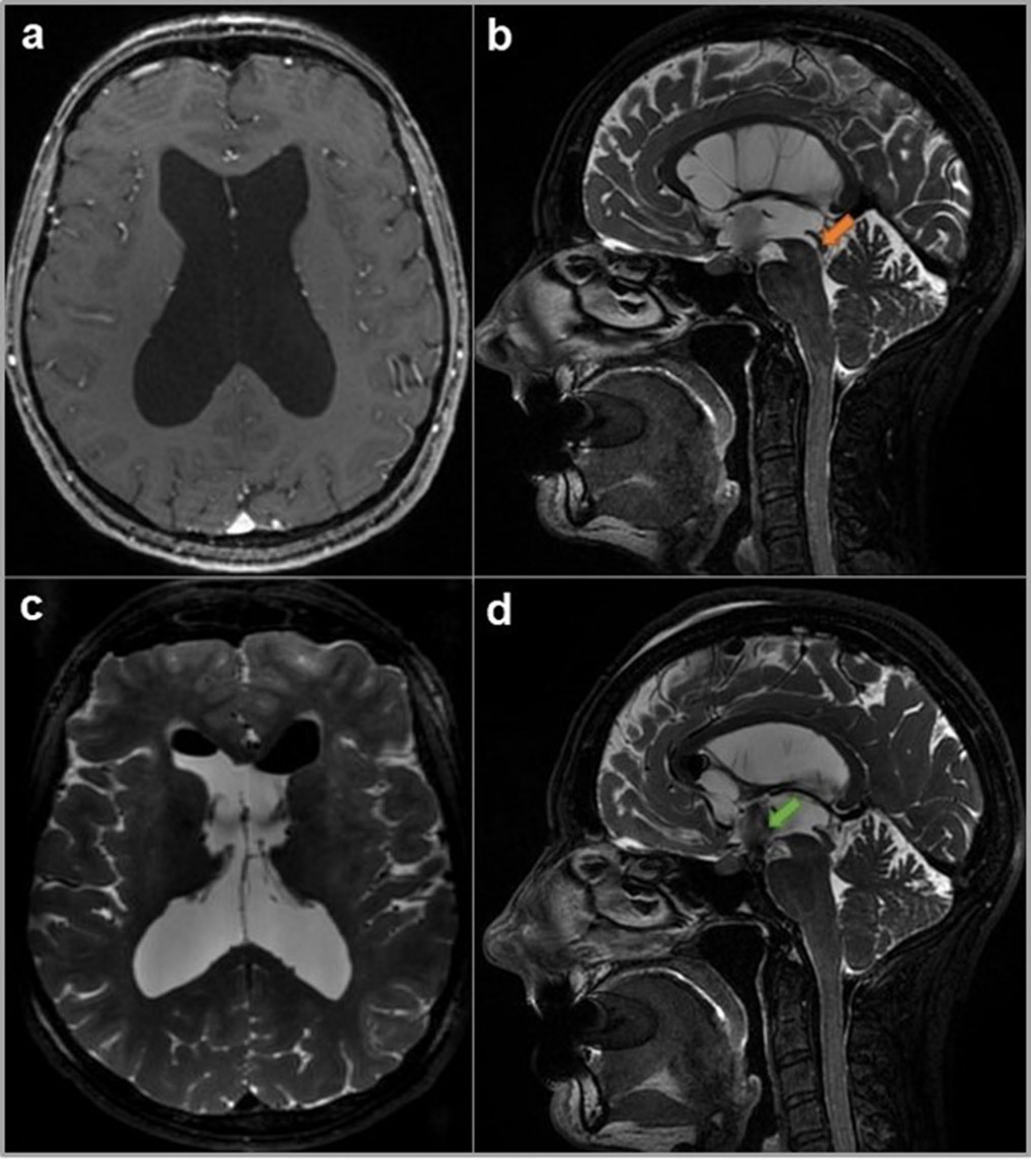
Pre- and post-operative MRI of an obstructive hydrocephalus treated by a combined CT imaging and robot-assistance endoscopic third ventriculocisternostomy. a Pre-operative axial gadolinium T1-weighted MRI revealed an enlargement of the lateral ventricles and a tri-ventricular hydrocephalus. b Pre-operative sagittal T2-weighted MRI demonstrate a superiorly narrowing of the aqueduct (*orange arrow*) with a fourth ventricle not dilated, o note the absence of flow-void signal intensity at the aqueductal level and the absence of trans-ependymal edema. c Post-operative axial T2-weighted MRI demonstrate a progressive reduction in ventricular size. d Post-operative sagittal T2-weighted MRI confirmed the correct fenestration in the floor of third ventricle and excellent flow passing through the ventriculostomy (*green arrow*)
